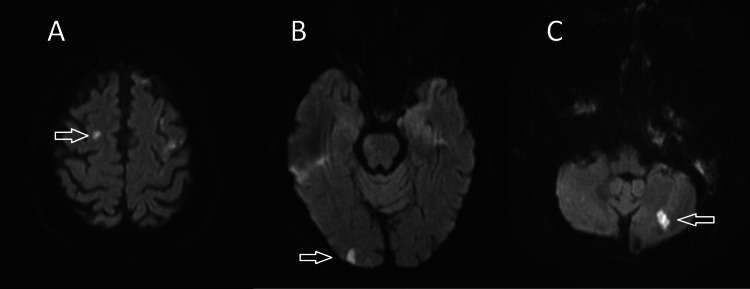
MRI head showing evidence of multiple small acute infarcts involving (A) right frontal lobe, (B) right occipital lobe, and (c) left cerebellar hemisphere (arrows).
